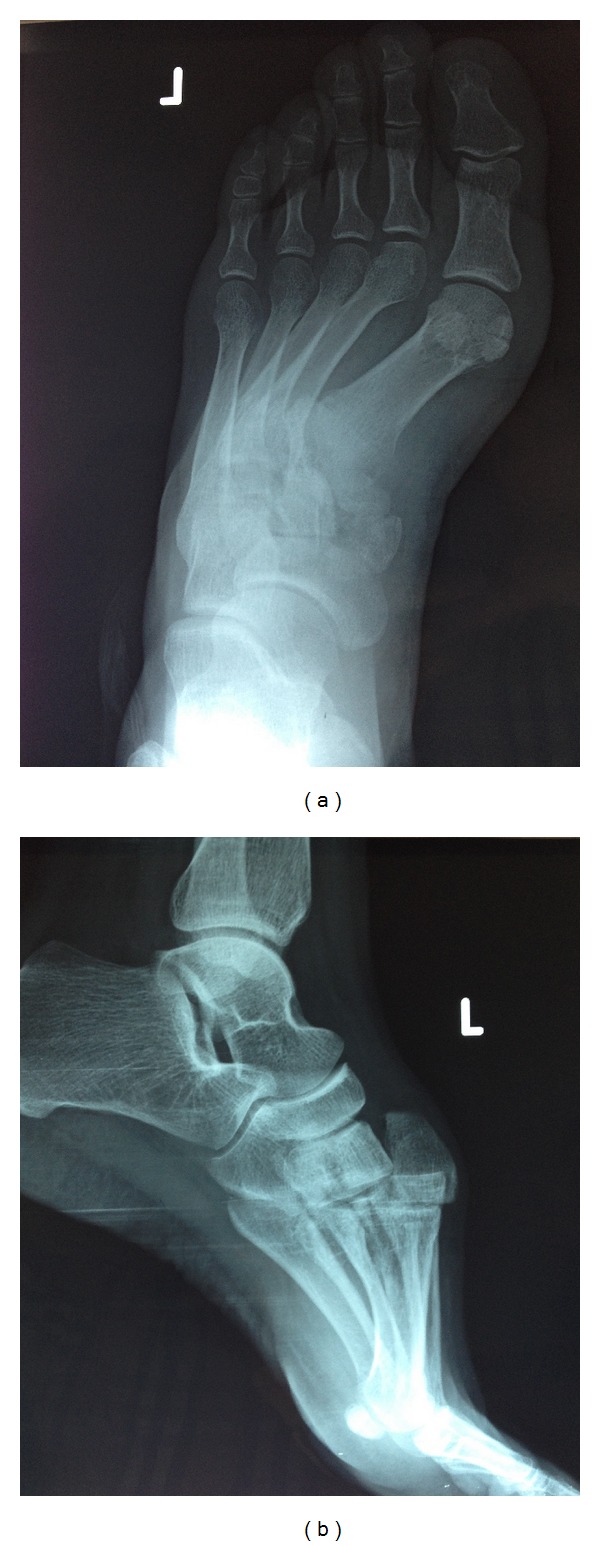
(a) Preoperative anteroposterior X-ray view. (b) Preoperative lateral X-ray view.
